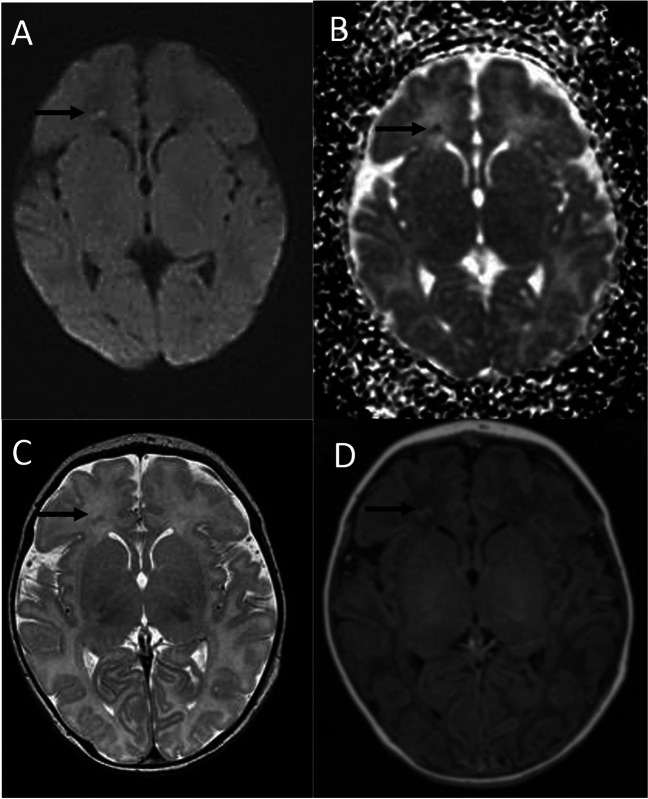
MRI of 6 days newborn performed brain MRI for suspected seizure activity (39 weeks GA) demonstrating frontal periventricular white matter focal injury characterized with restricted diffusion (arrow on A) with corresponding low ADC values (arrow on B). Note decreased T2 signal and high T1 signal (arrows on C and D)
